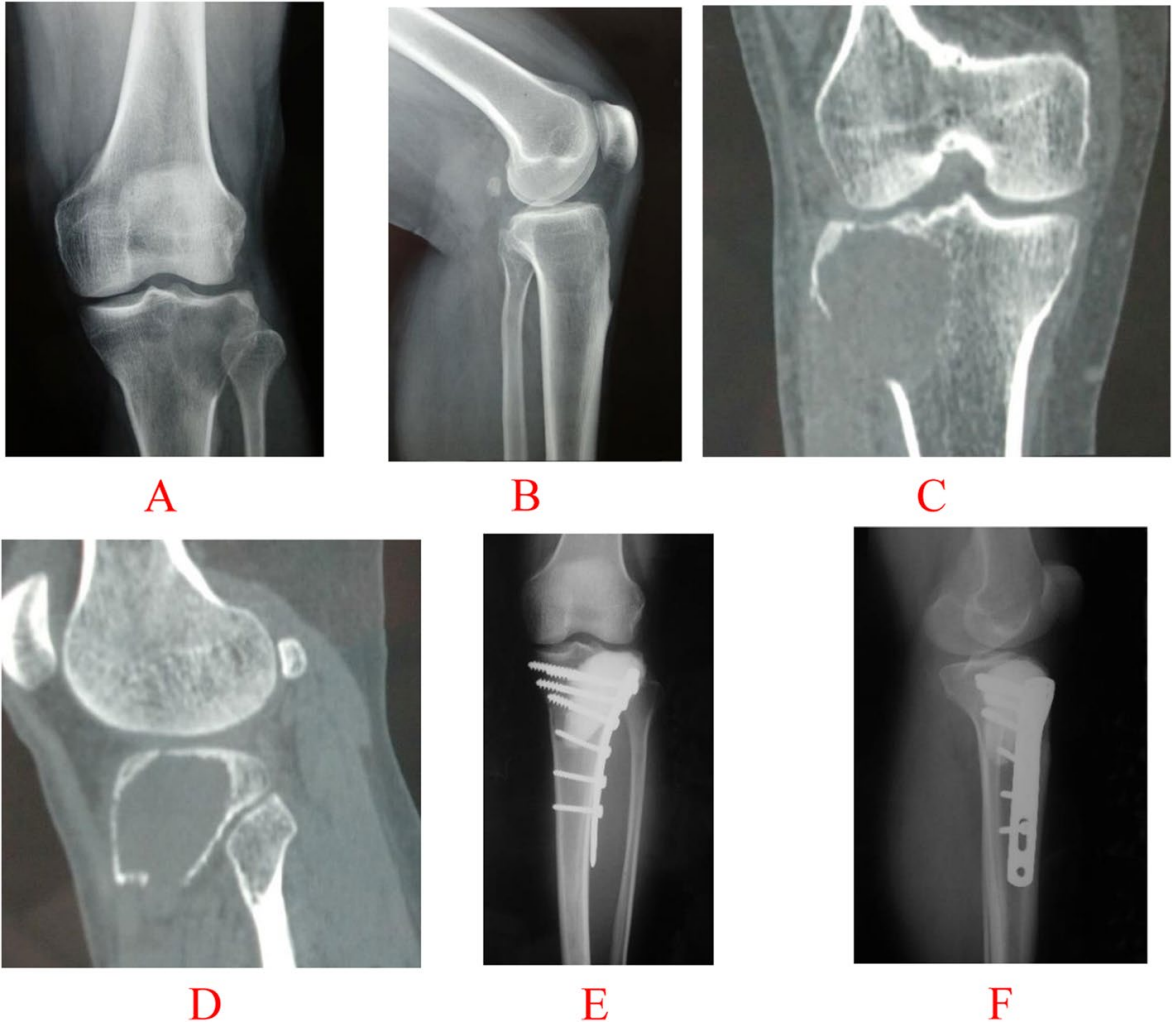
A 51-year-old male with a simple pathological fracture through GCT of the proximal tibia was treated with intralesional procedure. A, B Anteroposterior (AP) and lateral preoperative radiographs show the fracture is basically aligned. C, D Coronal and sagittal CT images show it involves extra-articular fracture and articular fracture with an broken joint surface, whose distance of fracture displacement less than 2 mm. E, F Anteroposterior (AP) and lateral postoperative radiographs made immediately after curettage, reconstruction with bone cement and internal fixation
